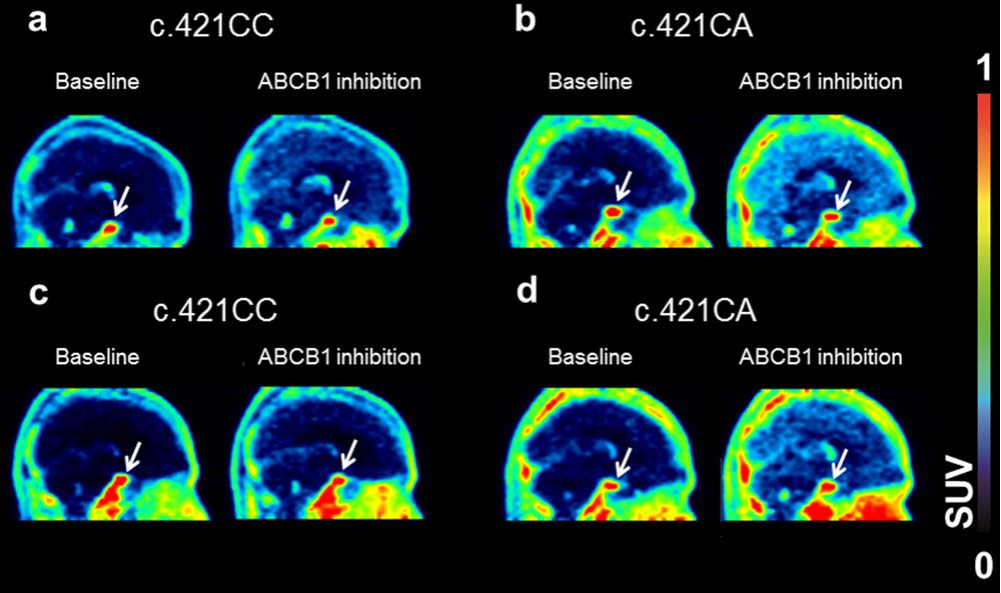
Sagittal PET summation images (0–60 min) for [^11^C]elacridar (a,b) and [^11^C]tariquidar (c,d) in c.421CC and c.421CA subjects for baseline scans and scans during ABCB1 inhibition. In b and d, an identical c.421CA subject is shown. Radioactivity concentration was normalized to injected radioactivity amount per body weight and expressed as standardized uptake value (SUV) and radiation scale is set from 0 to 1.0. Pituitary gland is labeled with white arrow.
